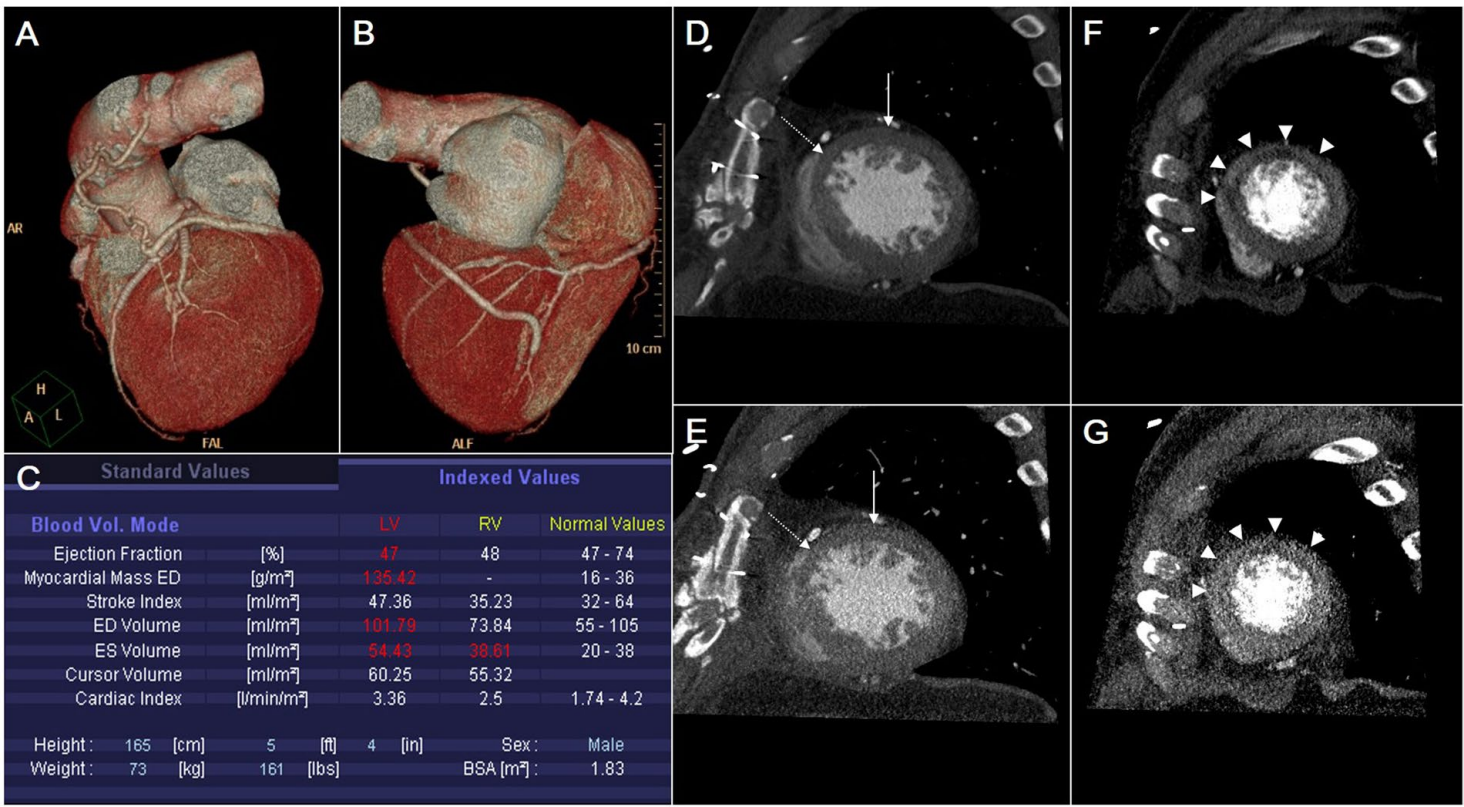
Functional analyses of the left ventricle (LV) using cardiac CT. (A) The patient underwent coronary artery bypass grafting (CABG). The left internal mammary artery (LIMA) was connected to the left anterior descending (LAD) artery. (B) Y-shaped graft using the saphenous vein connected to the posterior descending artery (PDA). (C) The ejection fraction of LV (LVEF) was 47%, representing mild systolic dysfunction. The LV chamber was enlarged to 102 mL/m^2^. The diastolic (D) and systolic (E) short-axis views of the mid-ventricular level show hypokinesia of segments 7 (arrow) and 8 (dotted arrow) of the LV myocardium. The diastolic (F) and systolic (G) short-axis views at the apical level show akinesia and subendocardial perfusion defects (arrowheads) at segments 13 and 14 of the LV myocardium. The wall motion score index (WMSI), measured using cardiac CT was 1.63, while the LVEF and WMSI, measured using early postoperative echocardiography, were 40% and 1.56, respectively. In the long-term follow-up echocardiography, the LVEF was improved to 68%, while the WMSI was aggravated to 1.62. The patient died after 4.7 year due to myocardial infarction.
